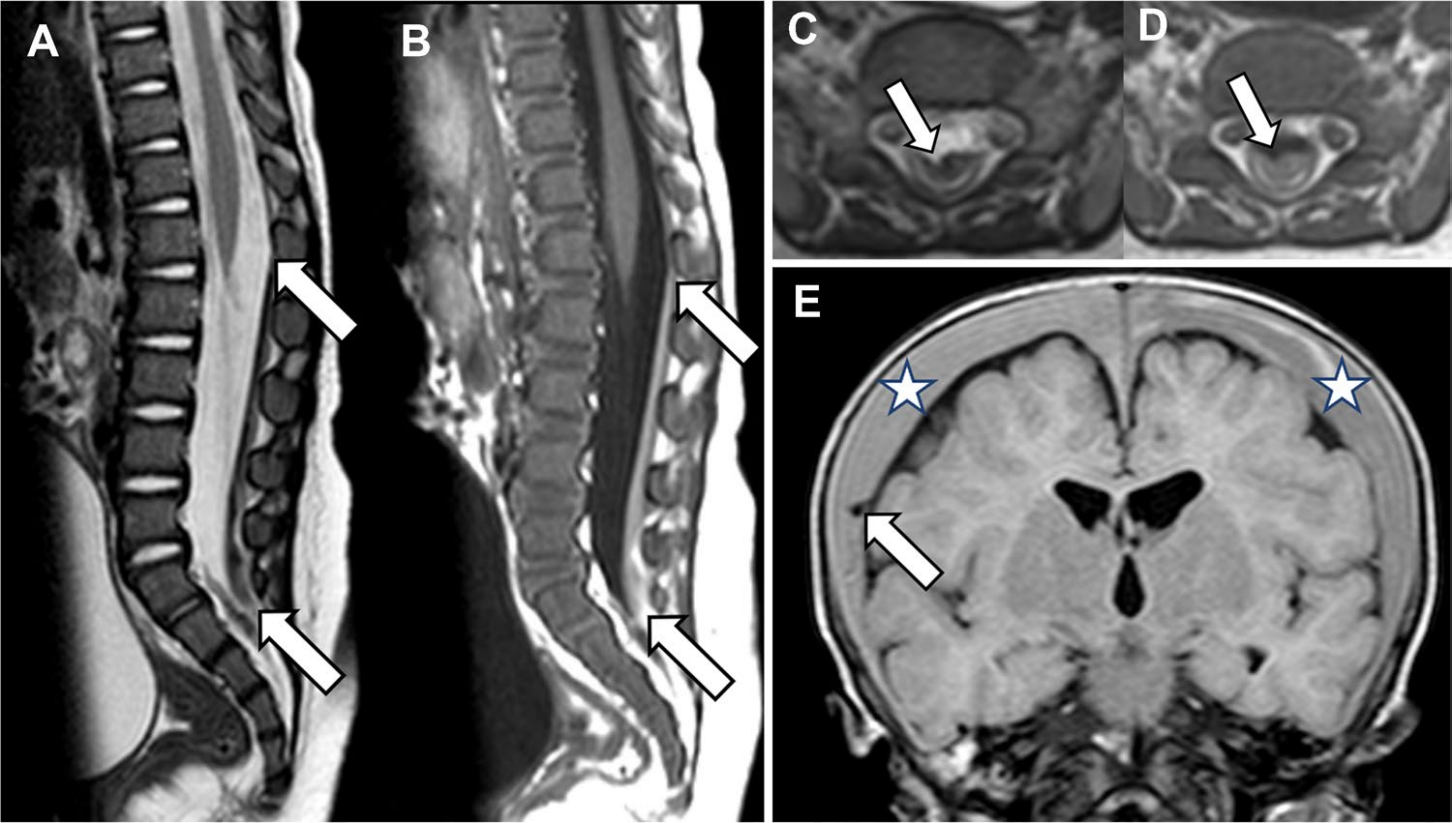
A 4-month-old male with abusive head trauma and thoracolumbar spinal subdural hemorrhage. Sagittal T2-weighted (A) and T1-weighted (B) MRI of the thoracolumbar spine demonstrates a T2-hypointense, T1-hyperintense dorsal SDH (*arrows*) extending from L1 to the distal thecal sac. Axial T2-weighted (C) and T1-weighted (D) MRI demonstrate a ventral indentation in the hematoma due to the presence of the midline dorsal septum, distinguishing it as subdural rather than epidural in location (the dorsal portion of an “inverted Mercedes Benz” sign). In this case, MRI of the cervical spine would have missed this critical finding. Coronal FLAIR brain MR image (E) shows bilateral convexity SDH (*asterisks*) and a torn or thrombosed bridging vein (*arrow*)
